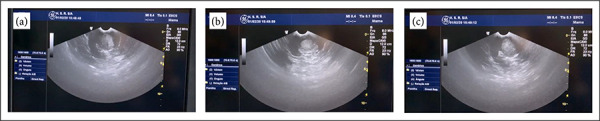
Endocavitary probe image projected onto the ultrasoundcart screen with visualization of the renal endophytic tumor.
